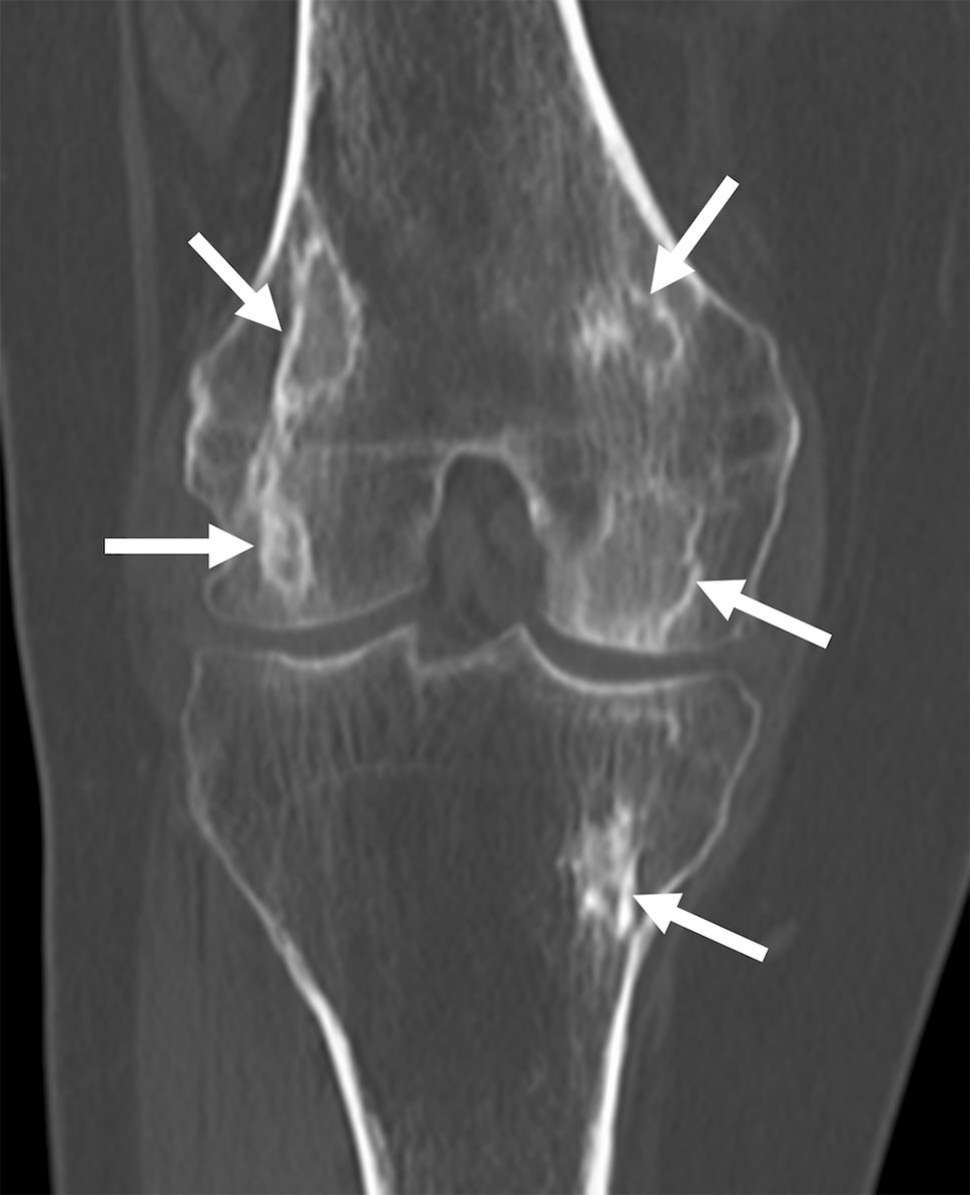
A 60-year-old female patient with sarcoidosis who had been treated with steroids for years. A reformatted CT coronal image shows multiple lesions with a serpiginous sclerotic border (arrows), which corresponds to lesions of AVN/bone infarction
